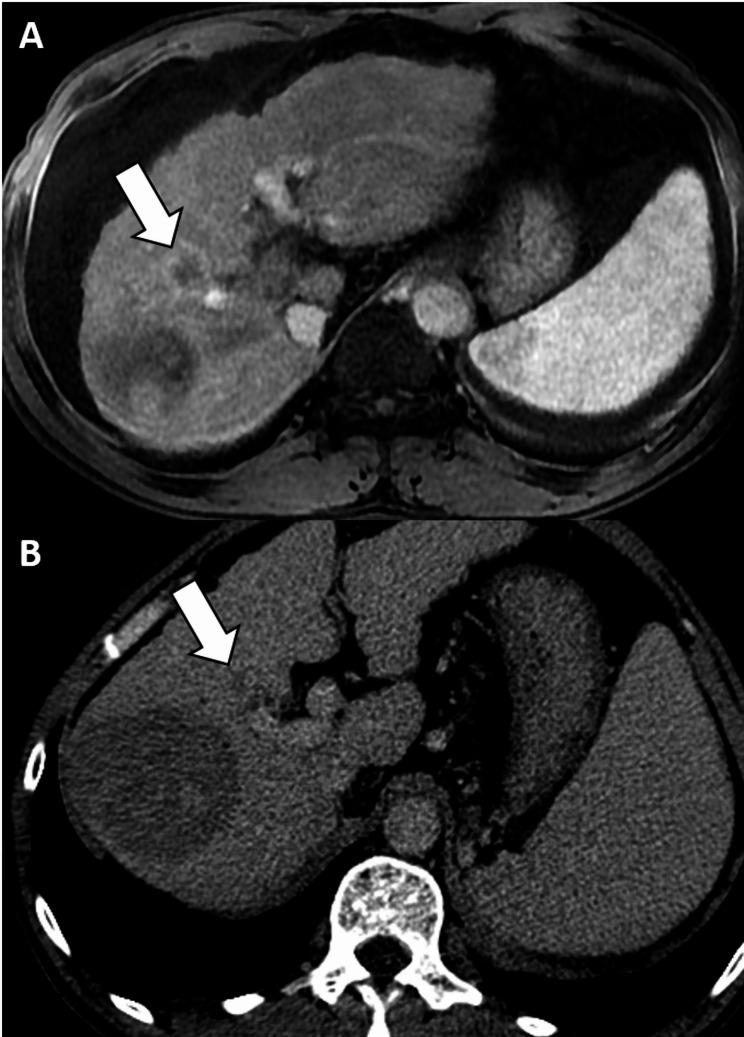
Nonspecific nature of threshold growth. (a) Arterial phase hepatobiliary contrast agent-enhanced axial MRI scan shows a 23 mm observation with rim hyperenhancement in segment 8 of the liver (arrow), which corresponds to LR-M. The same observation measured 15 mm on a delayed phase axial CT image obtained 5 months before (b), corresponding to threshold growth. There was no modification of LI-RADS category because the observation was initially categorized as LR-M. After liver explantation, cholangiocarcinoma was confirmed. Furthermore, there was a larger ablation zone at the posterolateral aspect of the observation on CT and MR images. CT, computed tomography; LI-RADS, Liver Imaging Reporting and Data System; MRI, magnetic resource imaging
